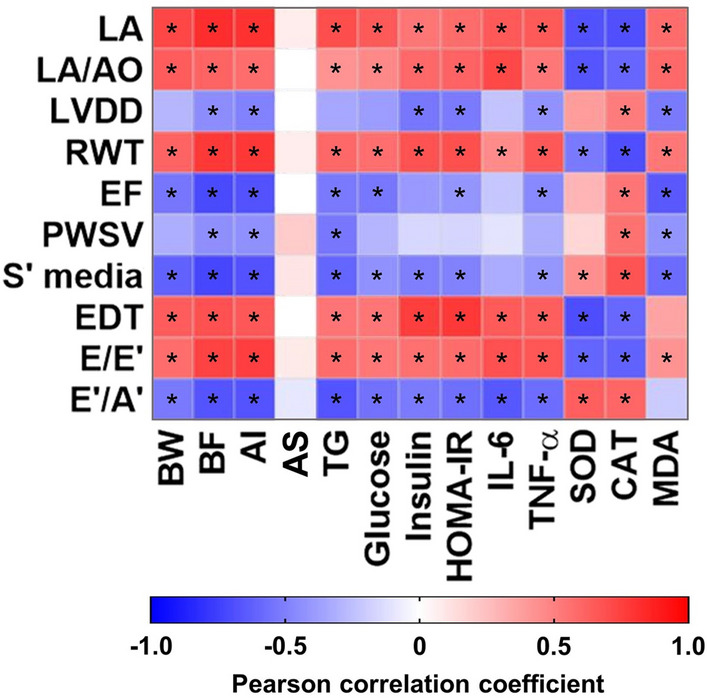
Correlation among structural and functional cardiac data and nutritional, metabolic, hormonal, inflammatory, and oxidative profiles visualized by a heatmap. *LA* left atrium diameter; *AO* aorta diameter; *LVDD* left ventricle (LV) diastolic diameter; *RWT* LV relative wall thickness; *EF* ejection fraction; *PWSV* posterior wall shortening velocity; *S’* tissue Doppler imaging (TDI) of mitral annulus systolic velocity; *EDT* E wave deceleration time; *E* early diastolic mitral inflow velocity; *E’* TDI of early mitral annulus diastolic velocity; *A’* TDI of late mitral annulus diastolic velocity; *BW* body weight; *BF* body fat; *AI* adiposity index; *AS* acid steatocrit; *TG* triglycerides; *IL-6* interleukin-6; *TNF-α* tumoral necrosis factor alpha; *SOD* superoxide dismutase; *CAT* catalase and *MDA* malondialdehyde. Data of all experimental groups were gathered and analyzed by GraphPad Prism 8 software using a 2-tailed Pearson’s correlation test. As shown by the color scale, the blue color indicates a negative correlation, while the red color indicates a positive correlation. The symbol * indicates a statistically significant correlation (*p* < 0.05)
